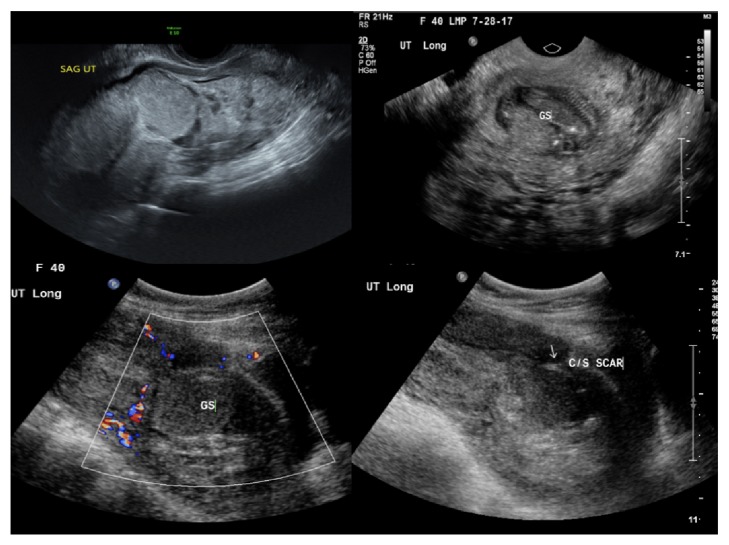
Sonographic findings when patient presented to the emergency room initially with the heterotopic gestation with a C-section scar ectopic containing fetal parts without discernible heart rate and an abnormal appearing intrauterine gestational sac in the lower uterine segment containing only low-level echoes.
